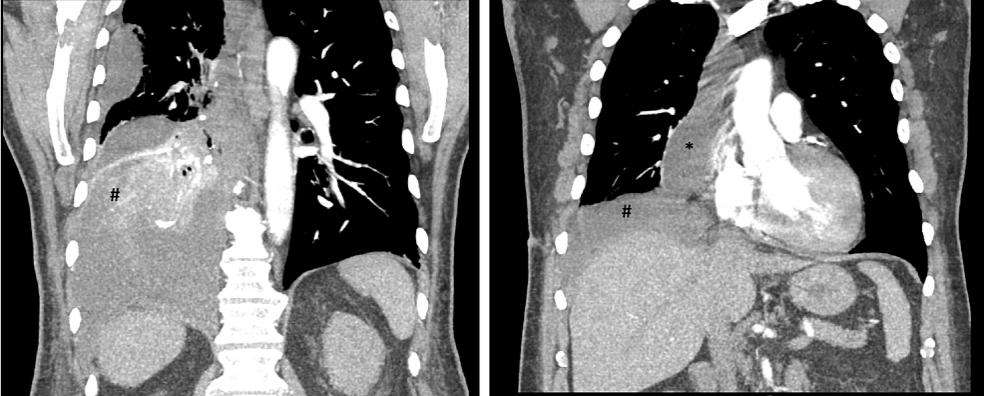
Coronal view of CT angiogram obtained on admission revealing a significant right pleural effusion with loculations (#) and right pericardial effusion (*) measuring up to 4.1 cm.
